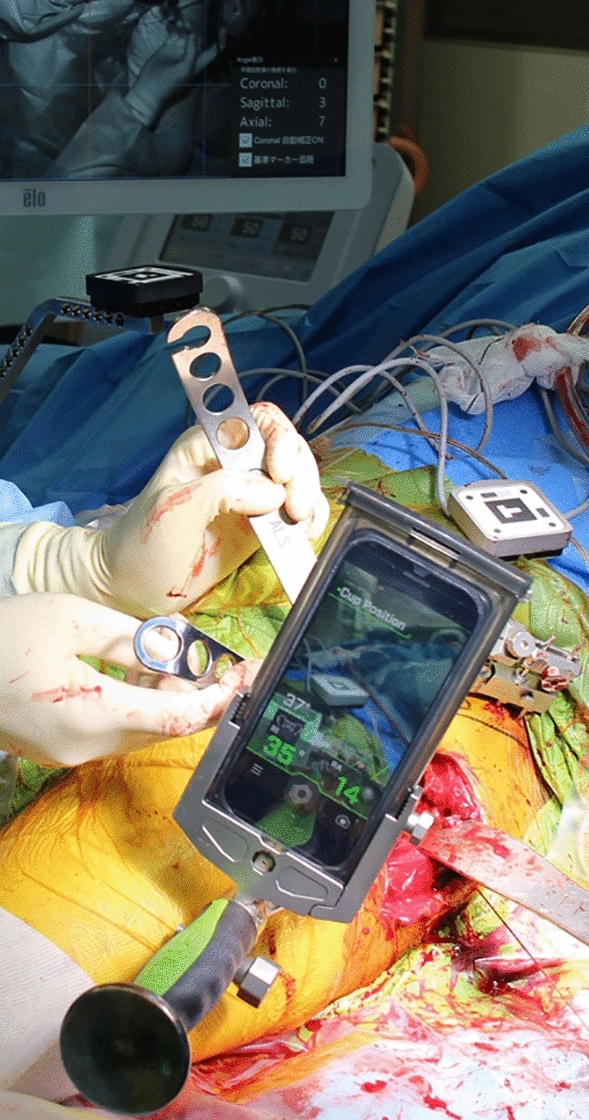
In the portable augmented reality (AR)-based navigation system, the smartphone is attached to the cup impactor. The smartphone recognizes the AR marker attached to the fixation pins at the pelvis. The display of the smartphone shows the radiographic inclination (RI) and radiographic anteversion (RA) of the cup.
